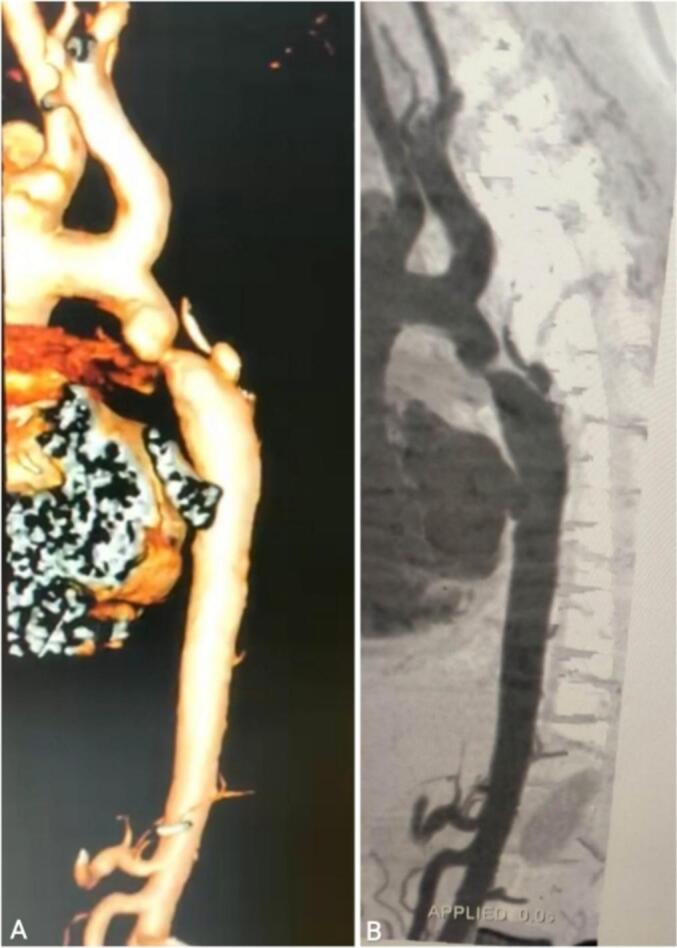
Two-dimensional (B) and three-dimensional (A) computed tomography scan imaging of coarctation of the aorta showing the discrete narrowing site at the aortic isthmus level.
